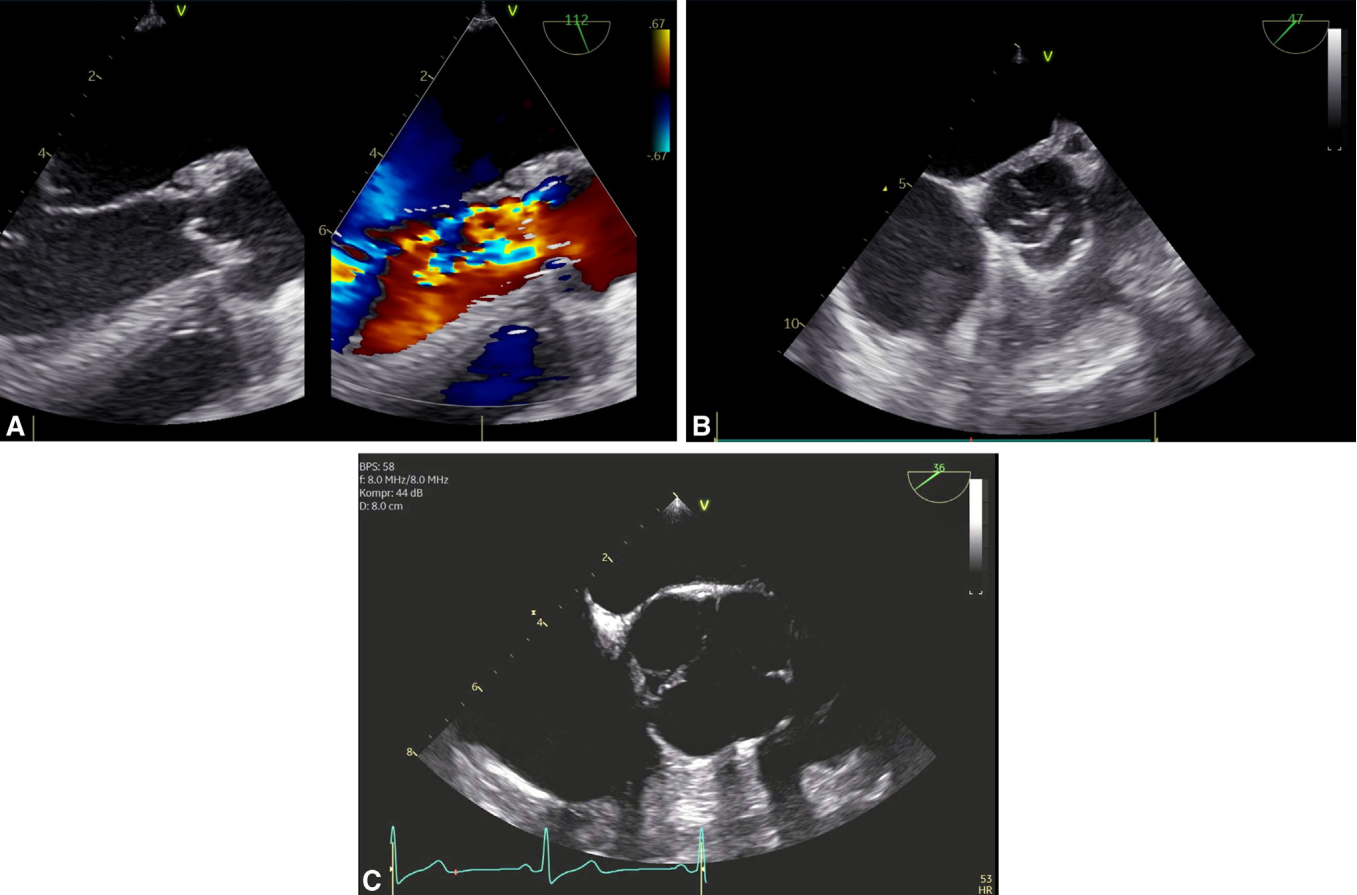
A, Intraoperative transesophageal long-axis view showing a larger conjoint new sinus compared to the other 2 sinuses with an asymmetric configuration after failed quadricuspid aortic valve (QAV) tricuspidization and severe aortic regurgitation. B, Intraoperative transesophageal short-axis view showing a larger conjoint new sinus compared to the other 2 sinuses with an asymmetric configuration after failed QAV tricuspidization. C, Intraoperative transesophageal short-axis view showing some asymmetry after failed QAV bicuspidization.
